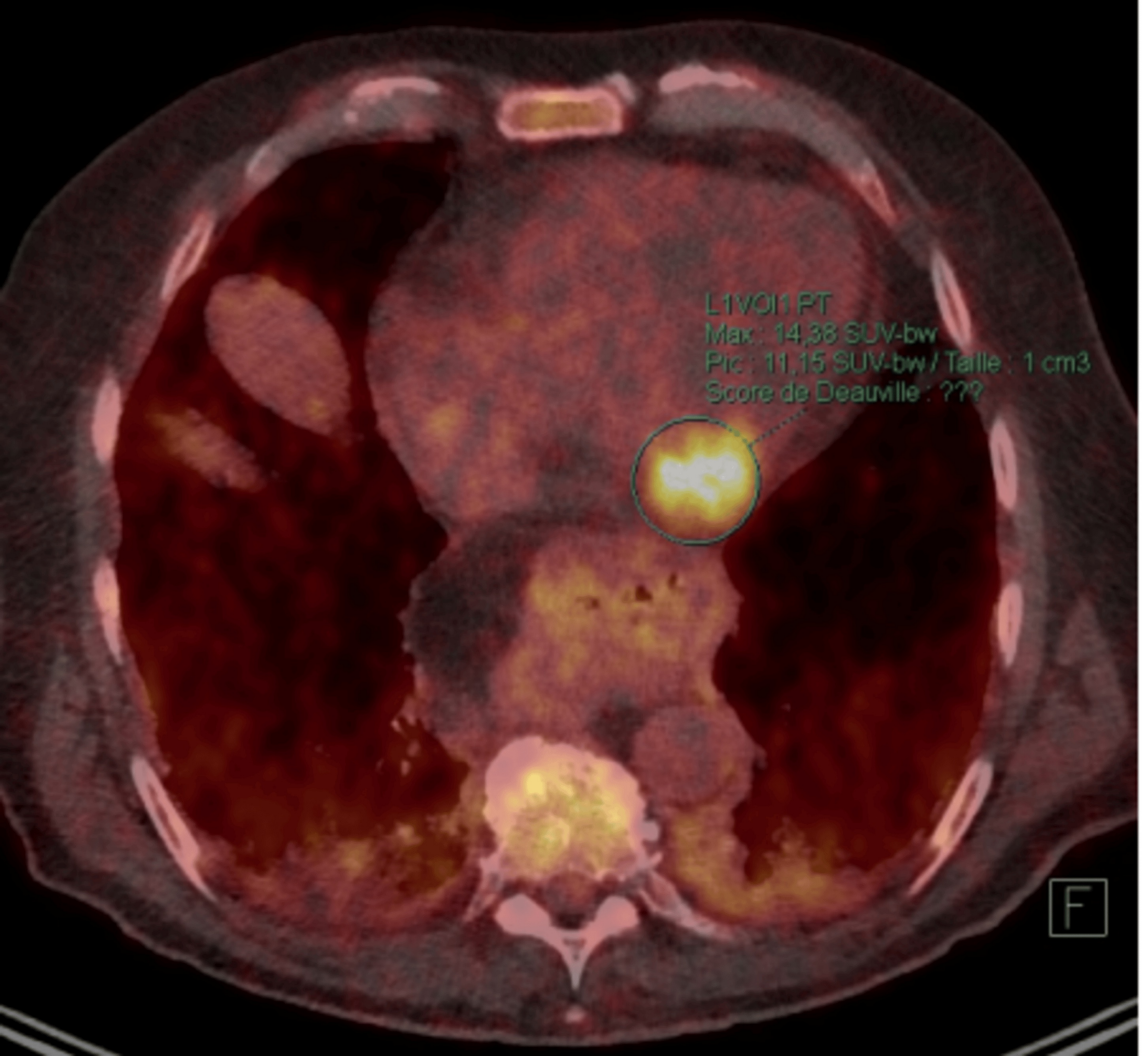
An 18FDG PET scan showing a hypermetabolism area in the left cardiac ventricle in axial view.
